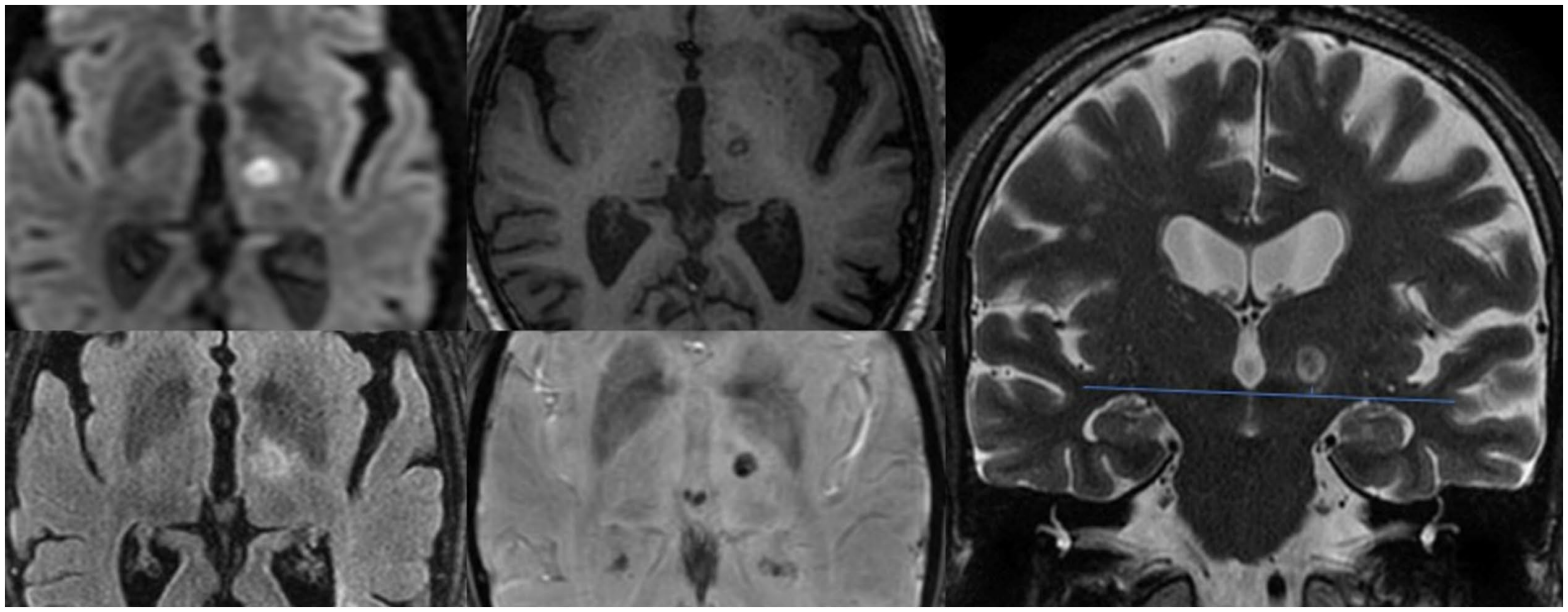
Evaluation of thalamotomy lesion axial plane diameter on DWI, FLAIR, T1 and SWI sequences, and caudal extension with respect to the AC-PC plane visualized on coronal T2 images.
